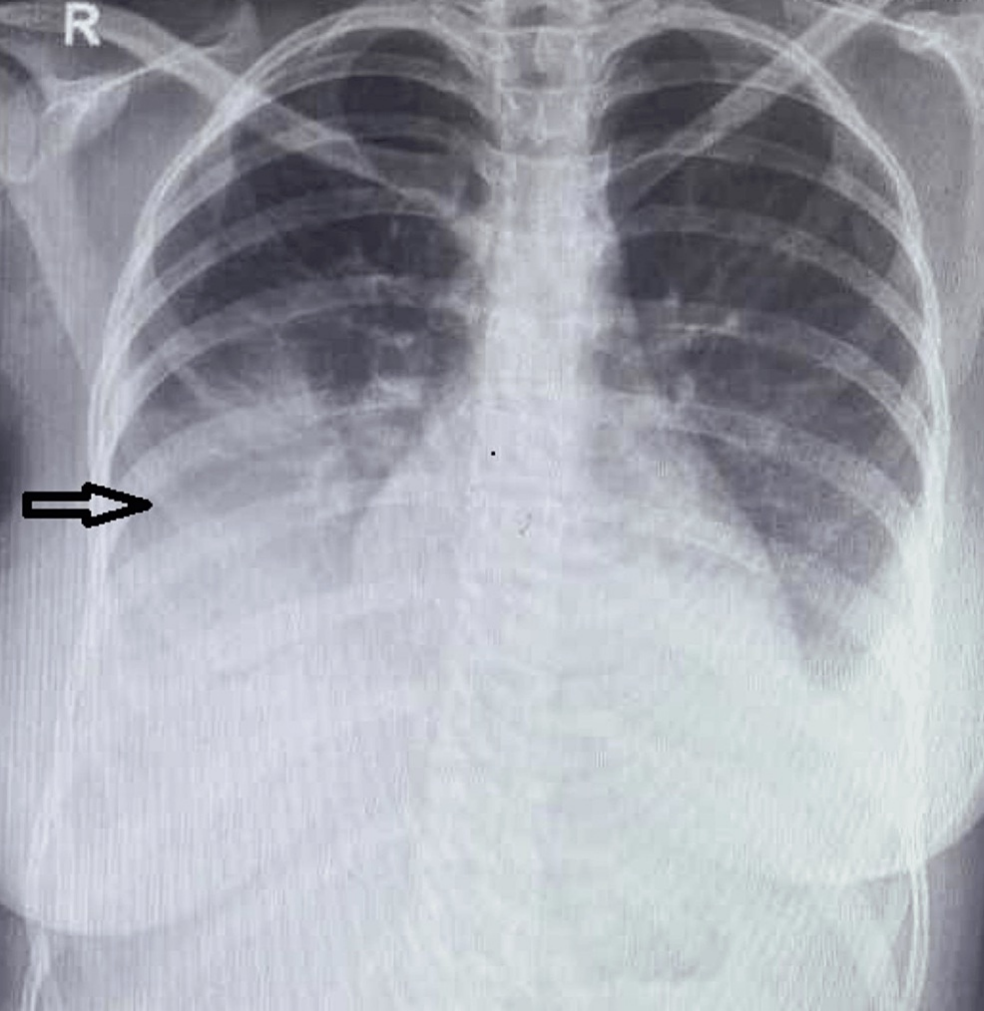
Repeat chest radiograph after three days, showing significant improvement in the opacities.
